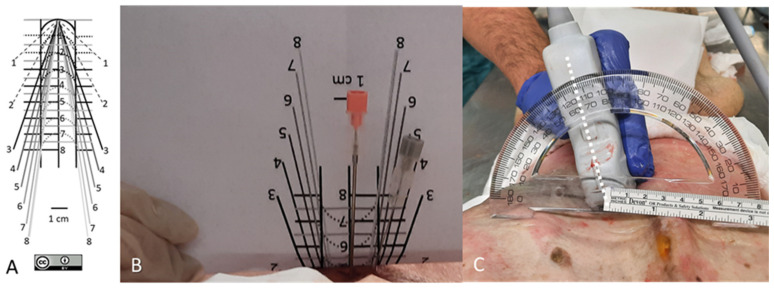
Representative images showing patient positioning and insertion guide. (A) Free visual guide for 1 cm paramedian approaches [20]. (B) The guide is folded based on the horizontal line corresponding to the skin–dural sac distance (d), as measured by ultrasound (5 cm in this specific case), and the needle is inserted 1 cm paramedially by following the oblique line corresponding to that distance. (C) The paramedian distance and the needle insertion angle following the axis of the ultrasound transducer (discontinuous line) may be individualized by searching for the best view of the interlaminar window.
